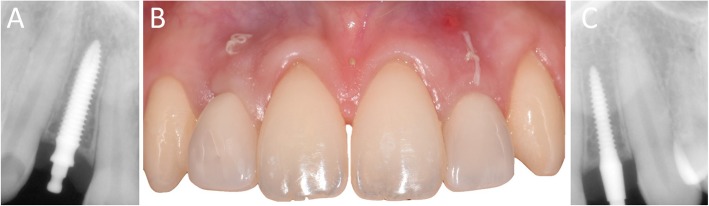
10-days post-surgical control, before sutures removal. a Right lateral incisor, radiographic control; b Frontal clinical view with provisional crowns in position; c Left lateral incisor, radiographic control
